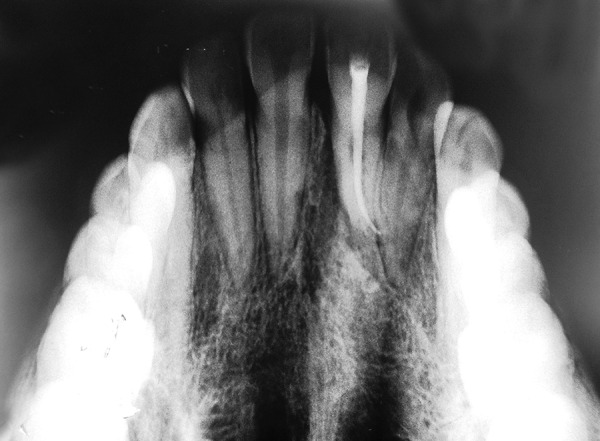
One-year postoperative occlusal radiograph is showing slight resorption on apex of tooth. It might damage the cemental surface at the time of extraction
